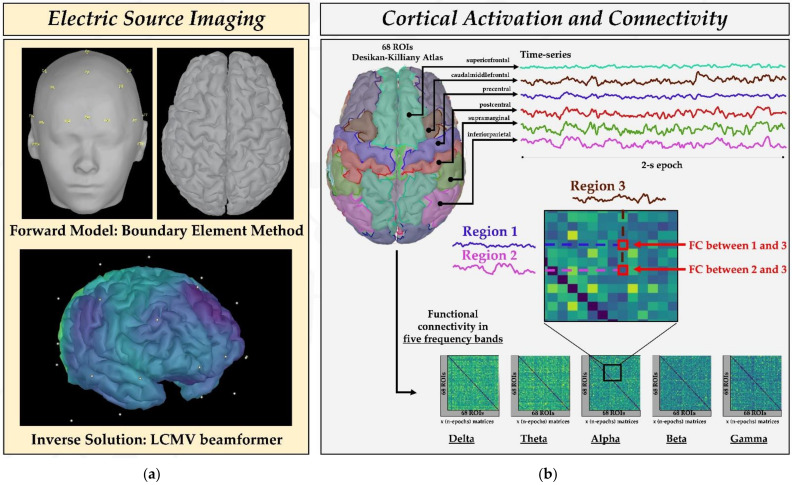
Source connectivity analysis. (a) To estimate the cortical activity from EEG data, we performed electric source imaging (ESI). A 3-layer boundary element method (BEM) was applied to solve the forward problem (i.e., compute the head model) after extracting the 3D head mask and cortical surface (top left) from each patient’s MRI. Electrodes were positioned on the head based on the 10–20 system. A linearly constrained minimum variance (LCMV) beamformer was used to solve the inverse problem, which generated a cortical activation map for each 2 s epoch (bottom left). (b) We estimated the activity of each cortical region of interest (ROI), obtaining a time series for each ROI. Functional connectivity between these regions was estimated for five frequency bands (δ (2–4 Hz); θ (5–7 Hz); α (8–12 Hz); β (13–29 Hz); γ (30–70 Hz)). Each element of the connectivity matrix indicates how much an ROI is connected to another in that 2 s epoch.
